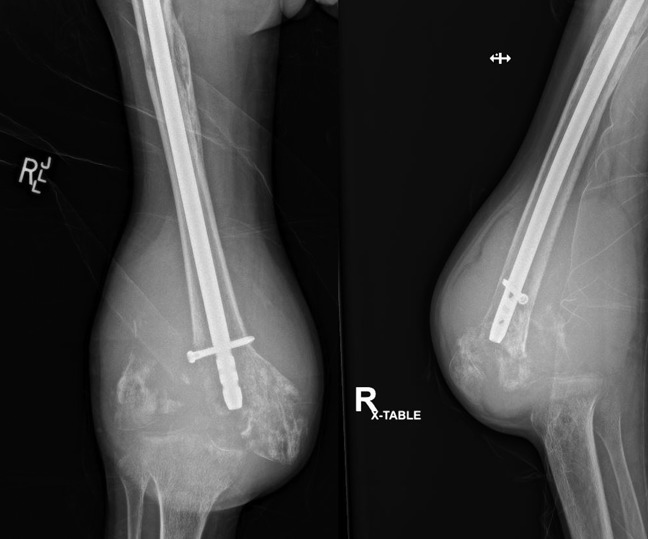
A radiograph at our initial clinical encounter demonstrating increased fracture displacement and osseous destruction of the distal right femur fracture with soft-tissue component.
